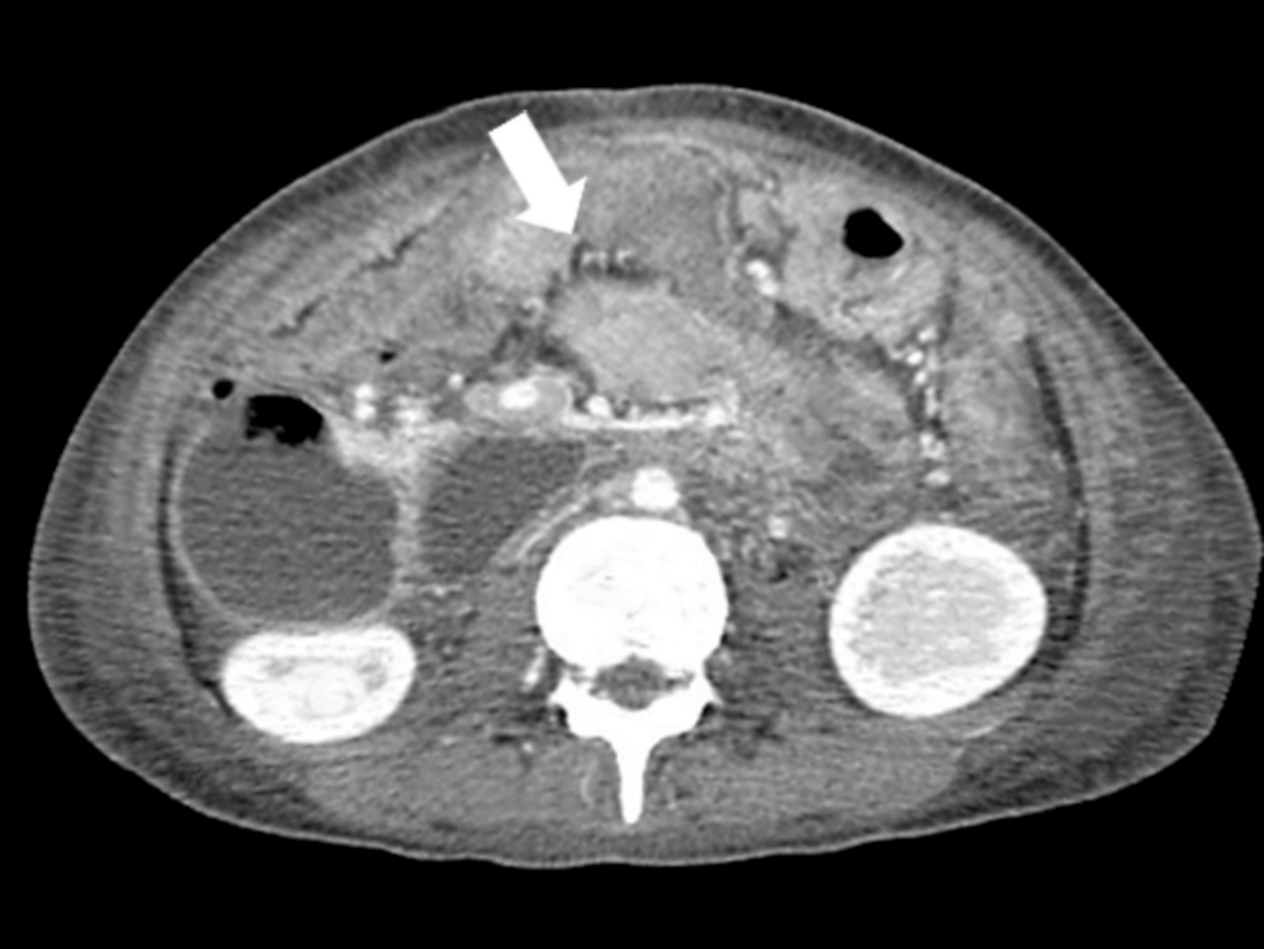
Contrast-enhanced CT scan demonstrating significant dilatation of the stomach and proximal duodenum due to extrinsic compression of the third portion of the duodenum. The arrow marks the site where the SMA compresses the duodenum against the abdominal aorta, consistent with SMA syndrome. SMA: superior mesenteric artery
